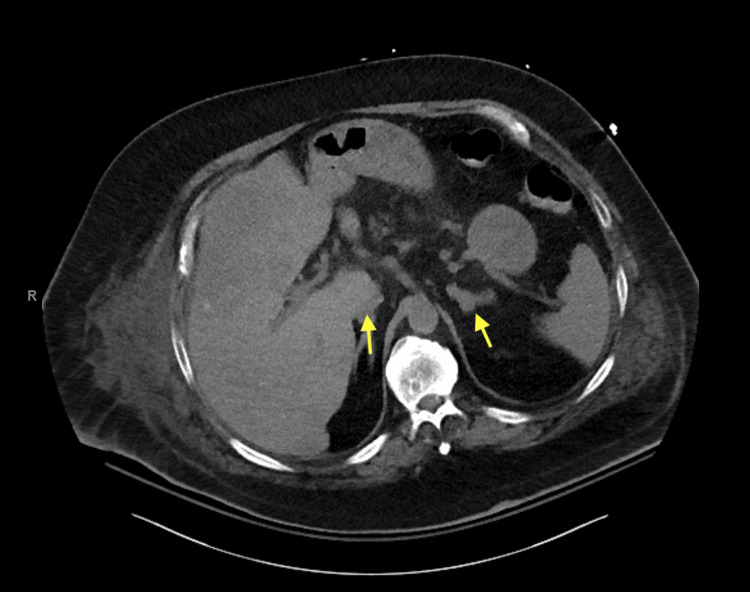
CT of the abdomen and pelvis demonstrating bilateral adrenal gland hypertrophy. CT: computed tomography
